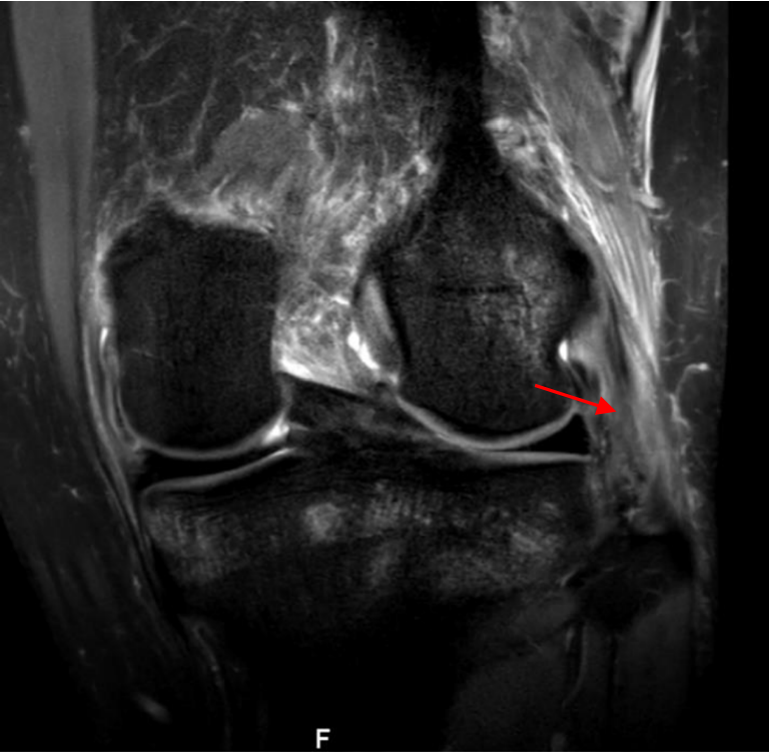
Partial mid-substance lateral collateral ligament tear (arrow) on coronal proton density fat saturation magnetic resonance imaging sequence (left knee)
